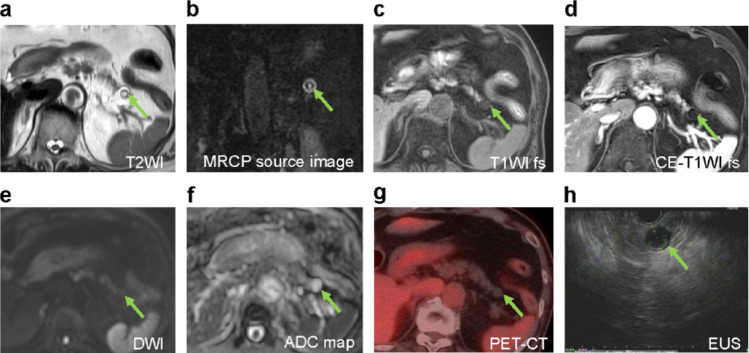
Non-enhancing lesion in an 83-year-old female a T2-weighted imaging showing a cystic lesion with a gravity-dependent nodular component (green arrow) exhibiting a target sign. b Magnetic resonance cholangiopancreatography source image demonstrating the characteristic target sign. c Fat-suppressed T1-weighted imaging showing hypointense signal. d Contrast-enhanced fat-suppressed T1-weighted imaging showing no enhancement. e Diffusion-weighted imaging showing low signal intensity. f Apparent diffusion coefficient map showing high values. g Positron-emission tomography-computed tomography showing no significant ^18^F-fluorodeoxyglucose uptake. h Endoscopic ultrasonography confirming the target sign appearance. Abbreviations: ADC, apparent diffusion coefficient; CE-T1WI, contrast-enhanced T1-weighted imaging; DWI, diffusion-weighted imaging; EUS, endoscopic ultrasonography; FDG, 18F-fluorodeoxyglucose; fs, fat suppression; MRCP, magnetic resonance cholangiopancreatography; PET-CT, positron-emission tomography-computed tomography; T1WI, T1-weighted imaging; T2WI, T2-weighted imaging
